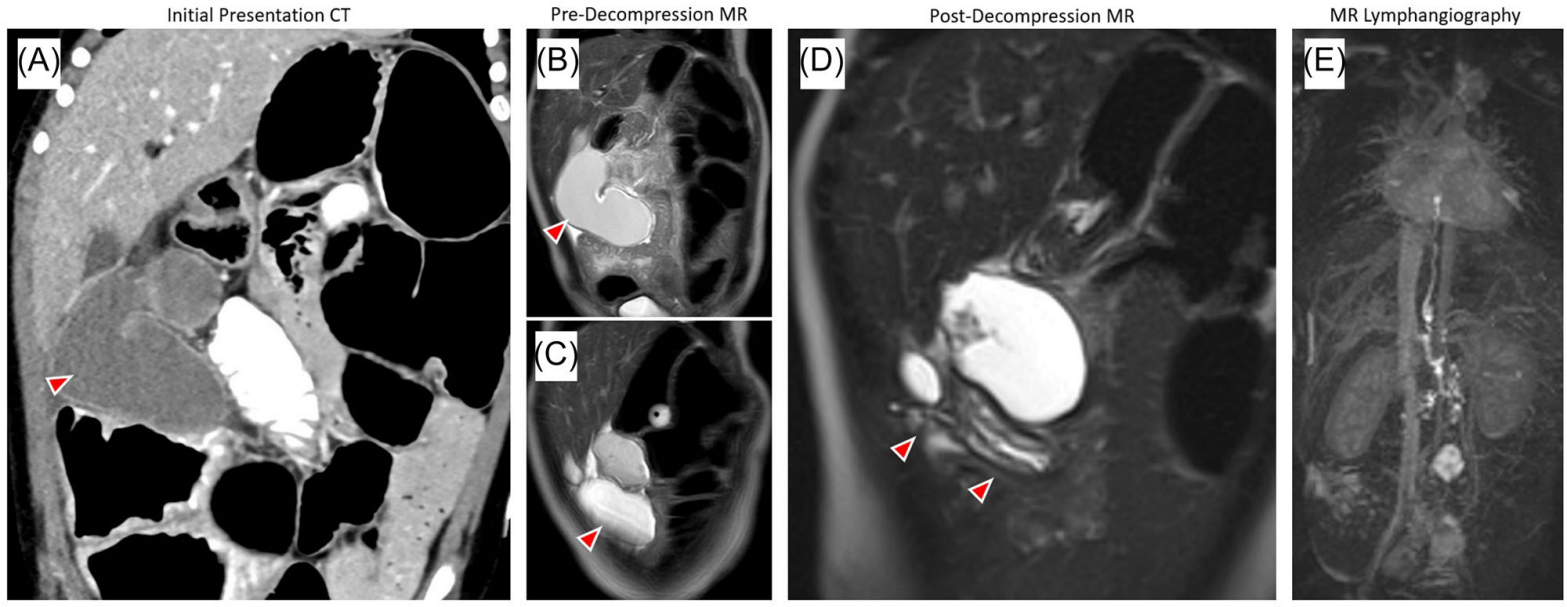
(A) Coronal computed tomography (CT) of the abdomen and pelvis with intravenous and oral contrast showing two fluid collections in the right abdomen; the lateral collection (arrow) is elongated and tubular. (B, C) Coronal T2‐weighted (T2W) magnetic resonance (MR) showing the fluid collections. (D) Coronal T2W MR as part of Dynamic MR Lymphangiogram (MRL) with a drainage catheter decompressing the lateral tubular collection (arrows), which showed mural stratification resembling gut layers (hypointense inner‐layer/mucosa, hyperintense mid‐layer/submucosa, hypointense external layer/muscularis, and serosa). Mural stratification only appeared after decompression. (E) Coronal T1‐maximum intensity Dynamic MRL image showing contrast in retroperitoneal lymphatic ducts and thoracic duct; no communication or leak observed.
